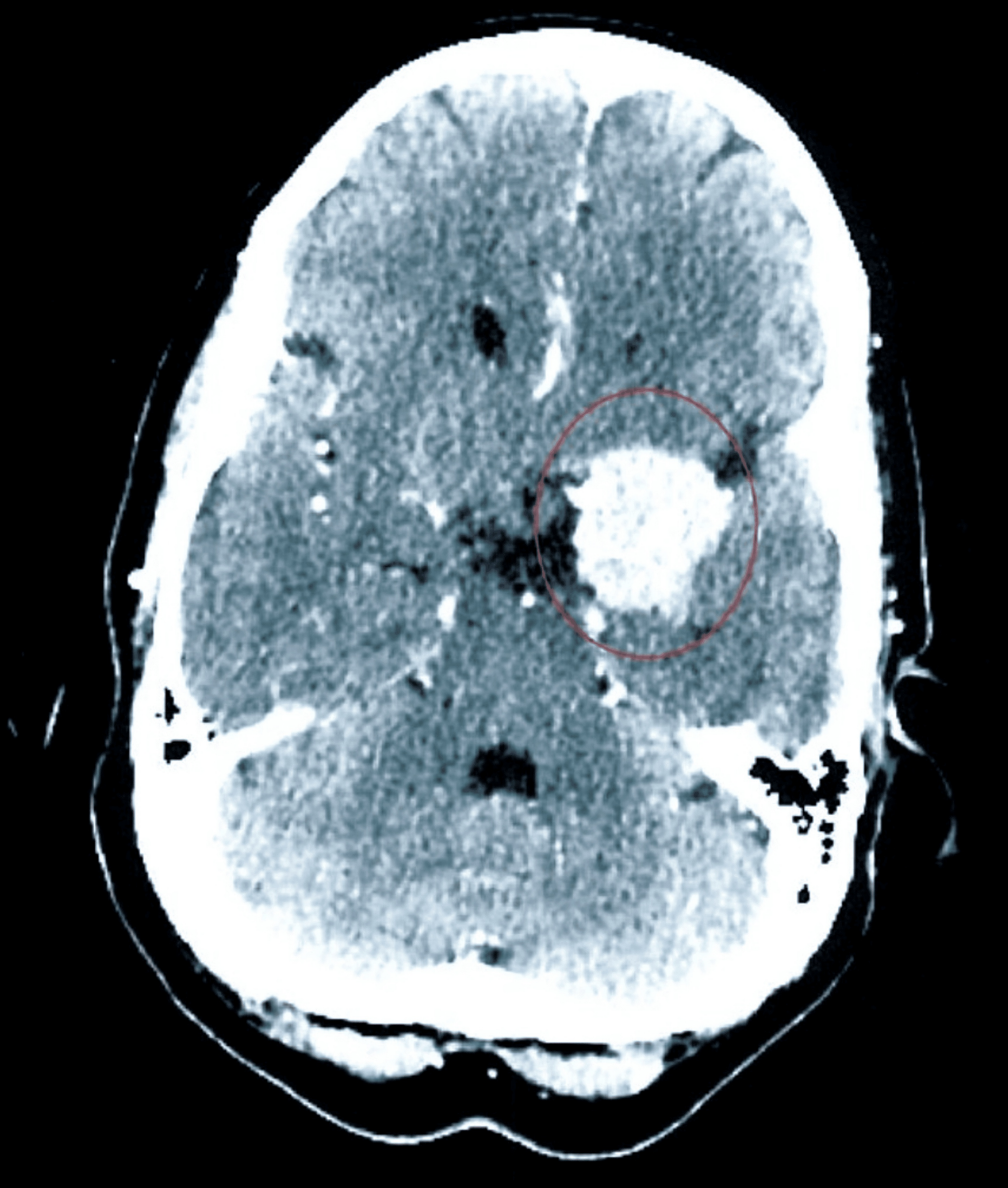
Pre-resection CT scan from October 2015 showing the tumor in the circled area
